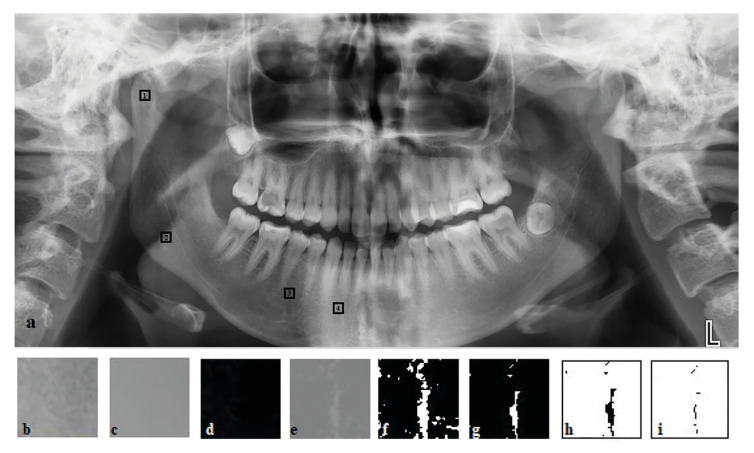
a: Panoramic radiograph with the five selected region of interest areas (ROIs); 1: the subcortical area of the condyle (ROI 1), 2: the supracortical area in the angulus (ROI 2), 3: the mesial area of the first molar (ROI 3), and 4: the apical area of the incisors (ROI 4) ; b: cropped and duplicated image; c: a gaussian blurred image ; d: a subtraction image ; e: an added 128 image ; f: binarization; g: erosion; h: dilation; and i: skeletonization.
